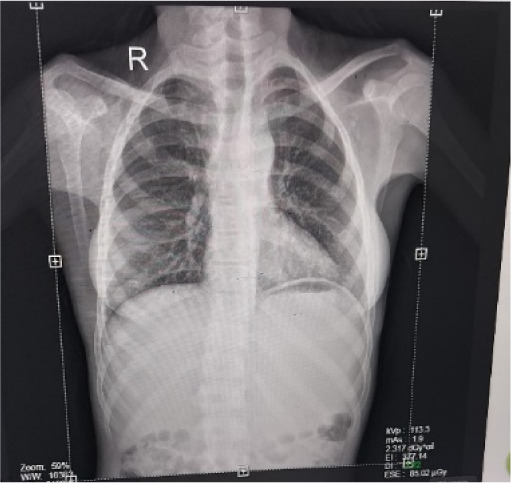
Chest X-ray of the patient showing increased concavity of the main pulmonary artery segment and syndesmosis of the right acromioclavicular segment
